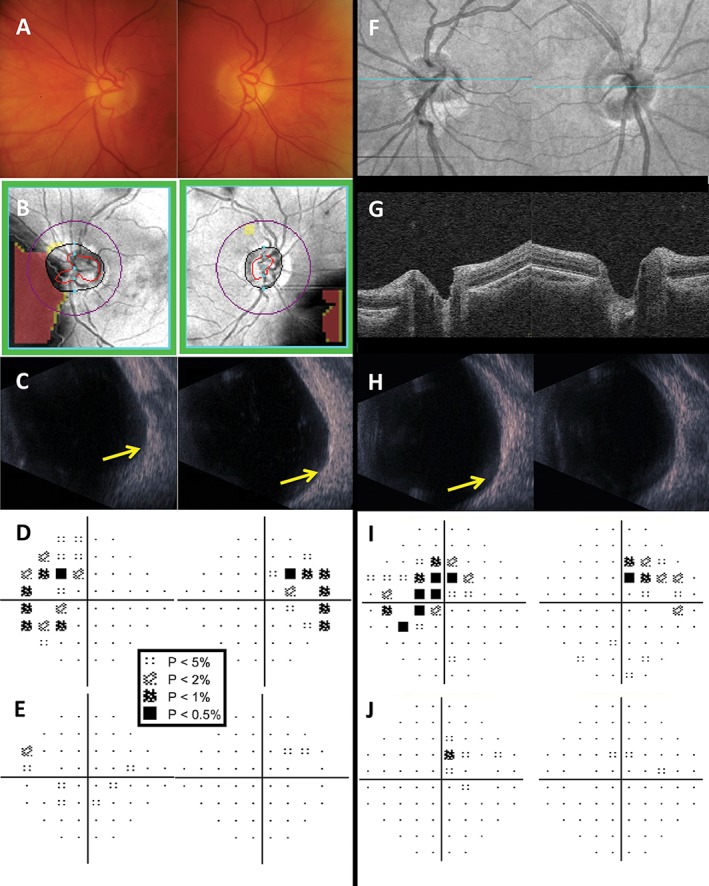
Two examples of patients with tilted disc syndrome. As per the convention of examining visual field results, left eye results are placed on the left and right eye results on the right; hence, the corresponding fundus photographs and optical coherence tomography (OCT) results are also placed on opposite sides to a typical instrument printout. (A) A 76‐year‐old man who was seen for assessment with a suspicious optic nerve head, which appeared tilted and obliquely inserted with clear situs inversus of the blood vessels typical of tilted disc syndrome. Cirrus OCT deviation map results (B) show obvious errors in segmentation of the nasal fundus, as expected of a coloboma in that region. B‐scan ultrasound along the horizontal axis in both eyes show an uneven curvature indicative of a posterior staphyloma in the region of the coloboma (C, yellow arrows). Conventional standard automated perimetry (SAP) testing (Humphrey Field Analyzer [HFA] 24–2 SITA‐Standard) showed a cluster of defects in the nasal region of both eyes which apparently respected the vertical midline (D). The addition of a further −3.25 D lens on top of the patient's near refraction almost completely eliminated the defect, by refocusing rays of light onto the more posteriorly displaced retina (E). Although there was some depression of the nasal, out‐of‐focus portion of the visual field, this did not reach statistical significance. (B) A 27‐year‐old woman with no neurological complaints but progression of myopia in the left eye. Fundoscopic examination showed a more obliquely inserted and tilted disc in the left eye (F, G; note that Cirrus OCT infrared images have been included in lieu of fundus photographs but show the same clinical picture) and subtle coloboma on B‐scan ultrasound (H). In comparison, the right eye showed only a slightly tilted disc (right hand side images). Conventional SAP testing (HFA 30–2 SITA‐Standard) revealed a cluster of defects predominantly in the superotemporal region, left more so than right (I). The addition of a further −3.25 D lens on top of the patient's refraction essentially eliminated the temporal visual field defect (J). Similar to the case shown in (A–E), there was some depression of the nasal, out‐of‐focus portion of the visual field; this did not reach statistical significance. A key for the greyscale levels of probability of normality for the deviation maps is shown as an inset.
